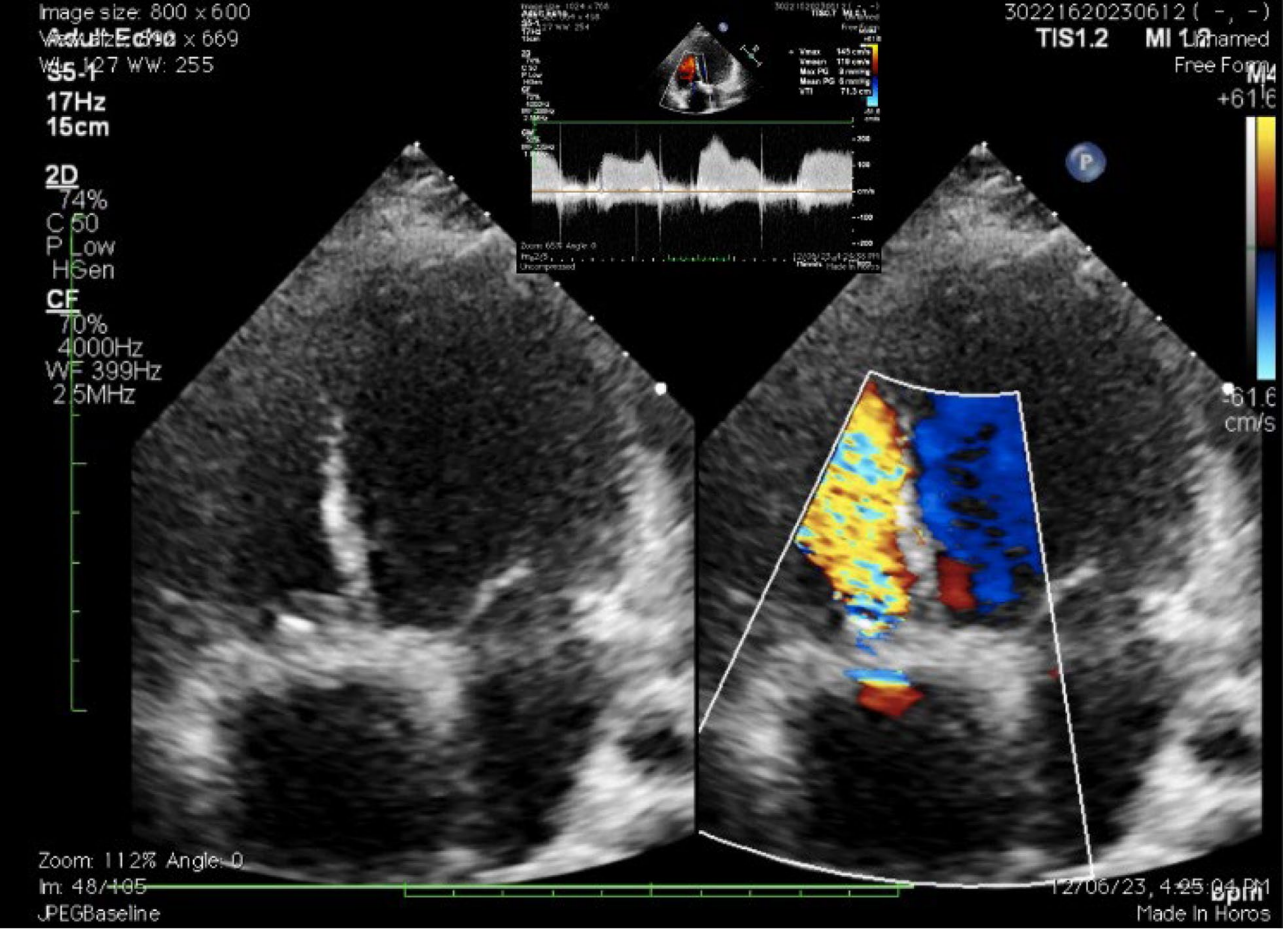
Echocardiogram showing apical 4 chamber view with color Doppler showing turbulent flow across tricuspid valve (off-set image showing continuous wave Doppler tracings with peak gradient of 8 mm Hg and mean gradient of 4 mm Hg)
